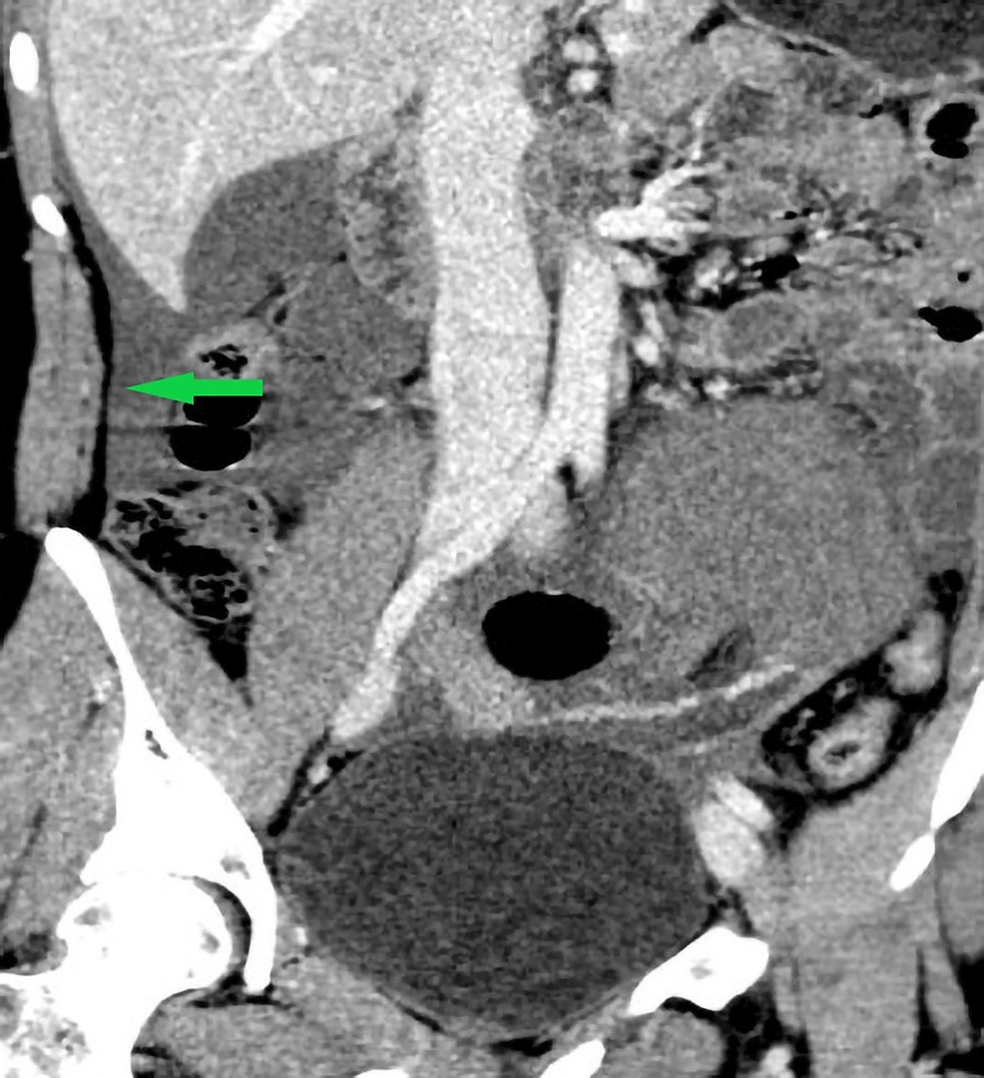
Coronal post-contrast CT demonstrates free fluid (green arrow).
